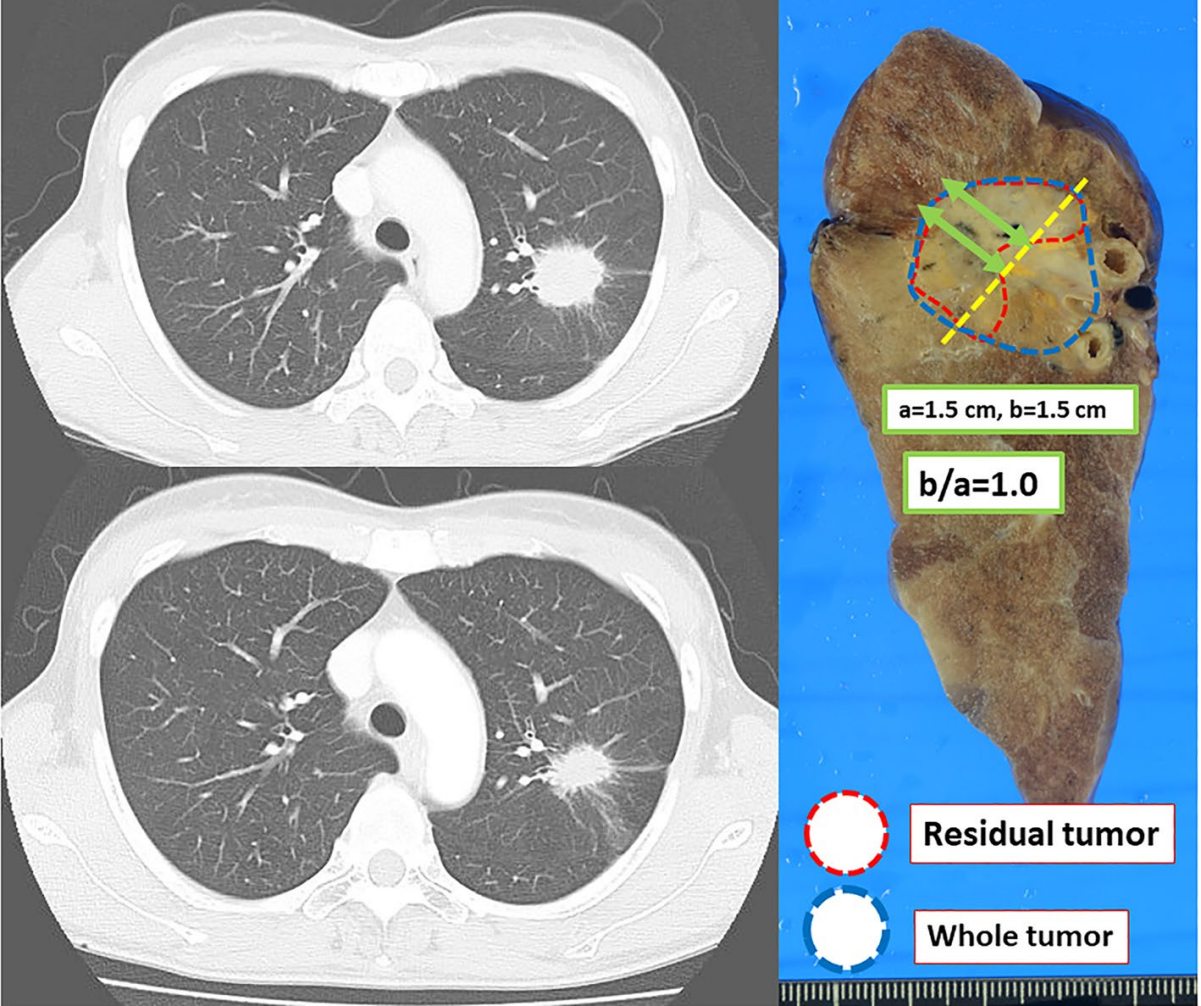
CT images before and after ICRT and the residual tumor location on surgical materials in adenocarcinoma. Residual tumor was located at the periphery side of primary tumor, of which b/a was 1.0
